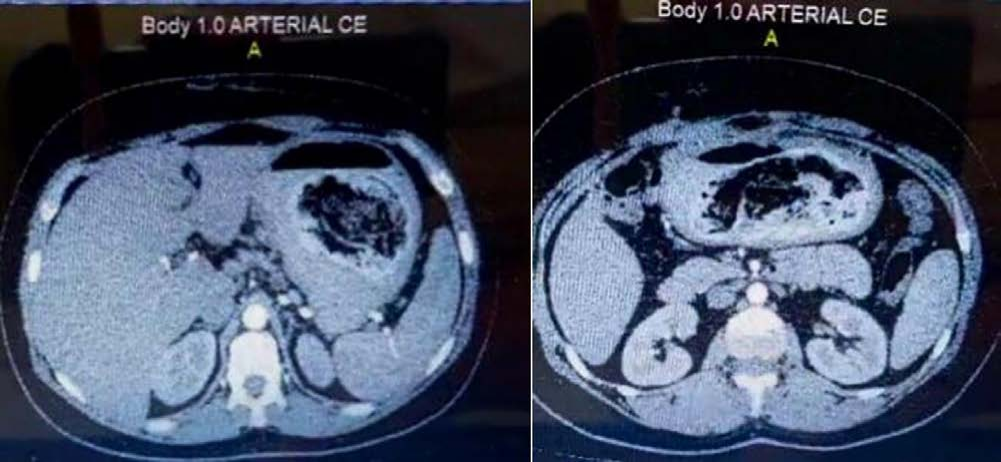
CT with contrast showcasing a large unattached heterogenous mass in the gastric lumen, with reactive diffuse and irregular thickening of the gastric mucosa, indicating a foreign body or malignancy. CT = computed tomography.
